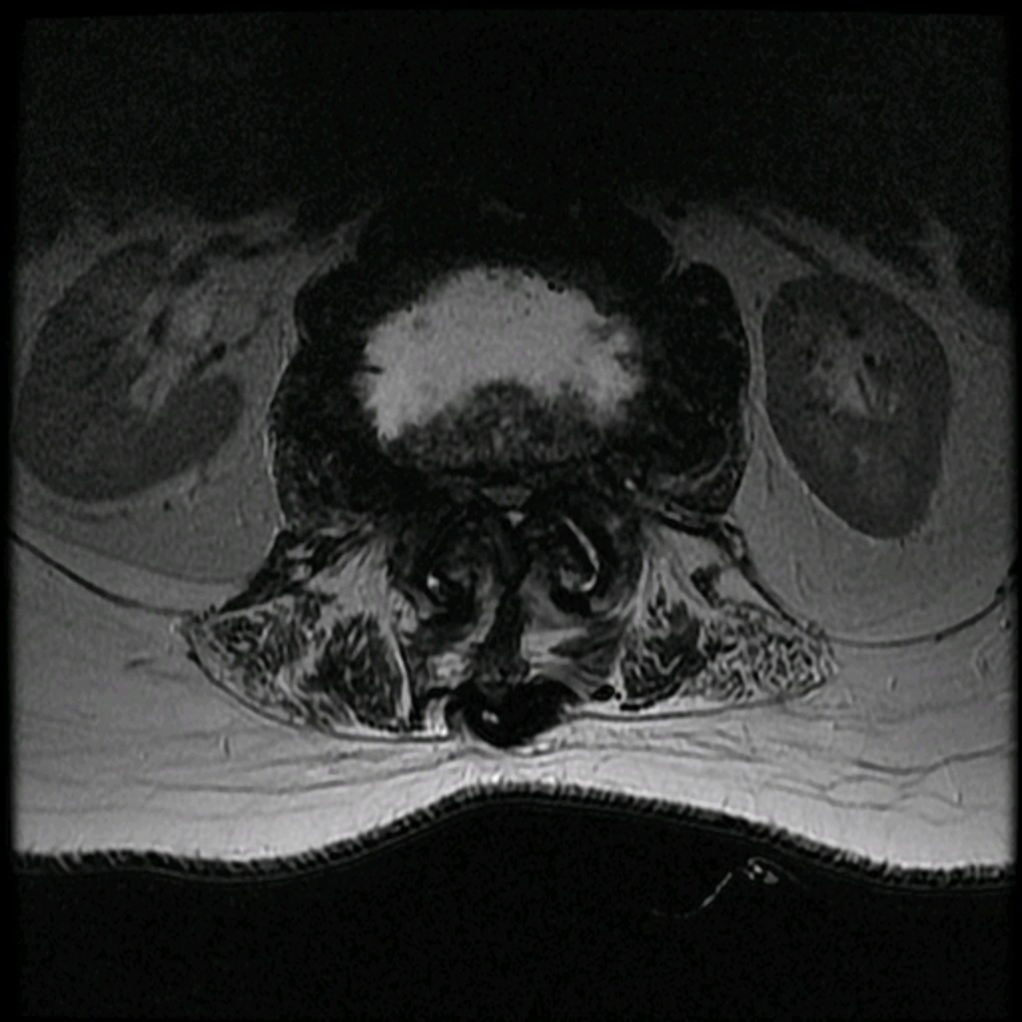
Axial T2 MRI This preoperative axial T2 MRI demonstrates severe spinal and neural foraminal stenosis at the L1-2 level.
